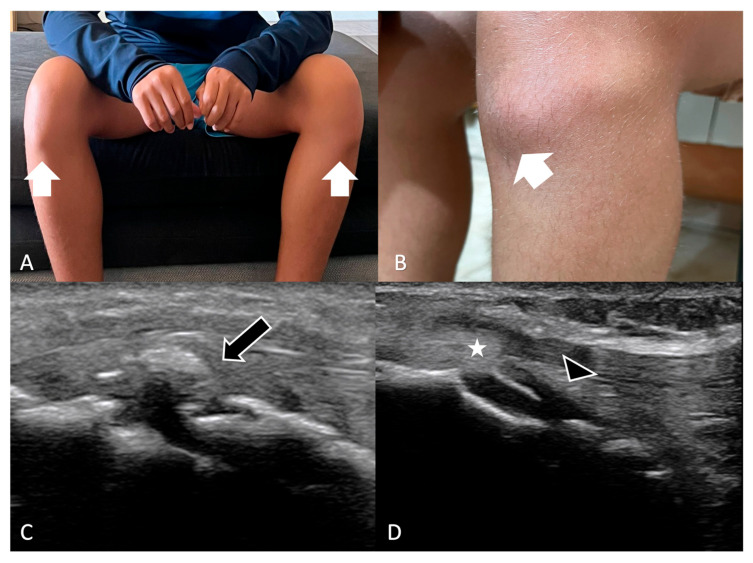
(A,B) Physical examination of a 12-year-old male reveals a bilateral deformity, presenting as bumps on the anterior aspect of both knees (white arrows), at the level of the tibial tuberosities. (C,D) Ultrasound examination shows irregularity and fragmentation of the anterior tibial apophysis growth nucleus (black arrows), thickening of the patellar tendon (white stars), and a small amount of fluid in the right intra-articular space (arrowhead).
